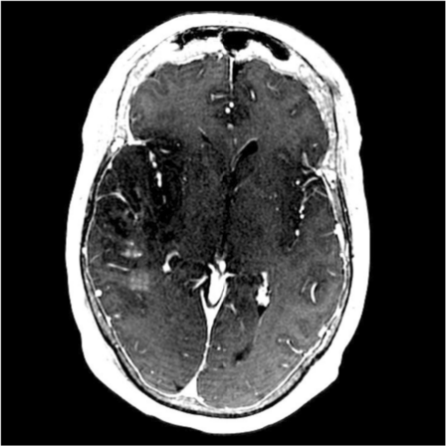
a Axial Gd-enhanced T1w MR images showing the first (top arrow) and second (bottom) BBB openings
